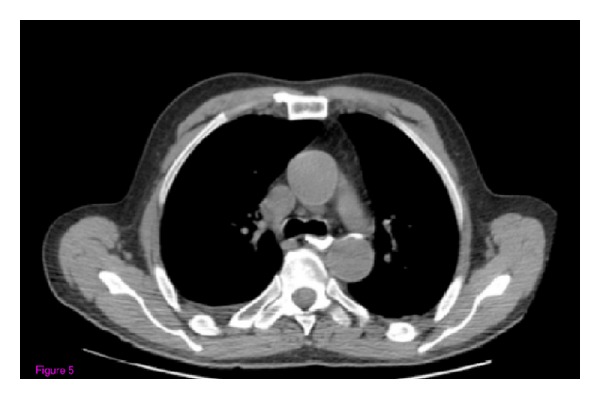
CT scan with gastrographin showing the contrast extravasation.
